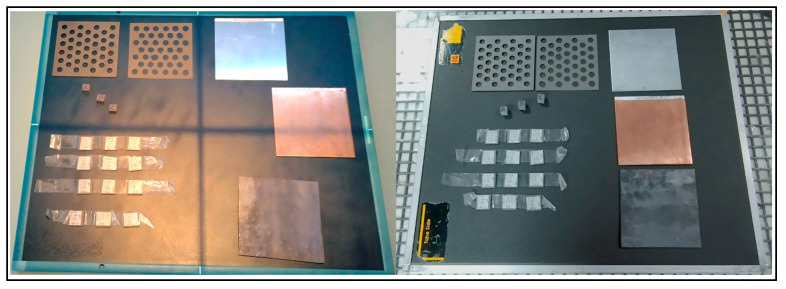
Samples of 12 slabs prepared with variation of printing parameters distributed in the order indicated in Table 1 (lower left corner), together with copper, aluminum, and lead filters (right) arranged in digital chassis for evaluation of kilovoltage radiography (left image) and 6 MV scintigraphy (right image).
